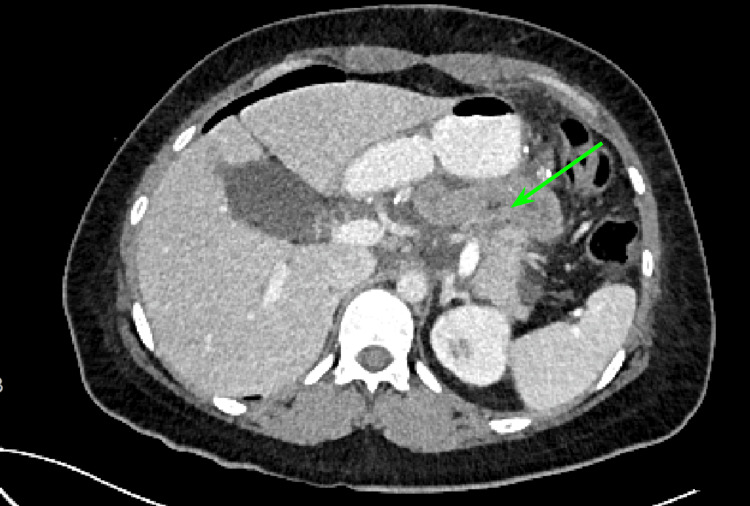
Abdominal CT scan. Edema and stenosis of the jejunum-jejunum anastomosis (green arrow).
